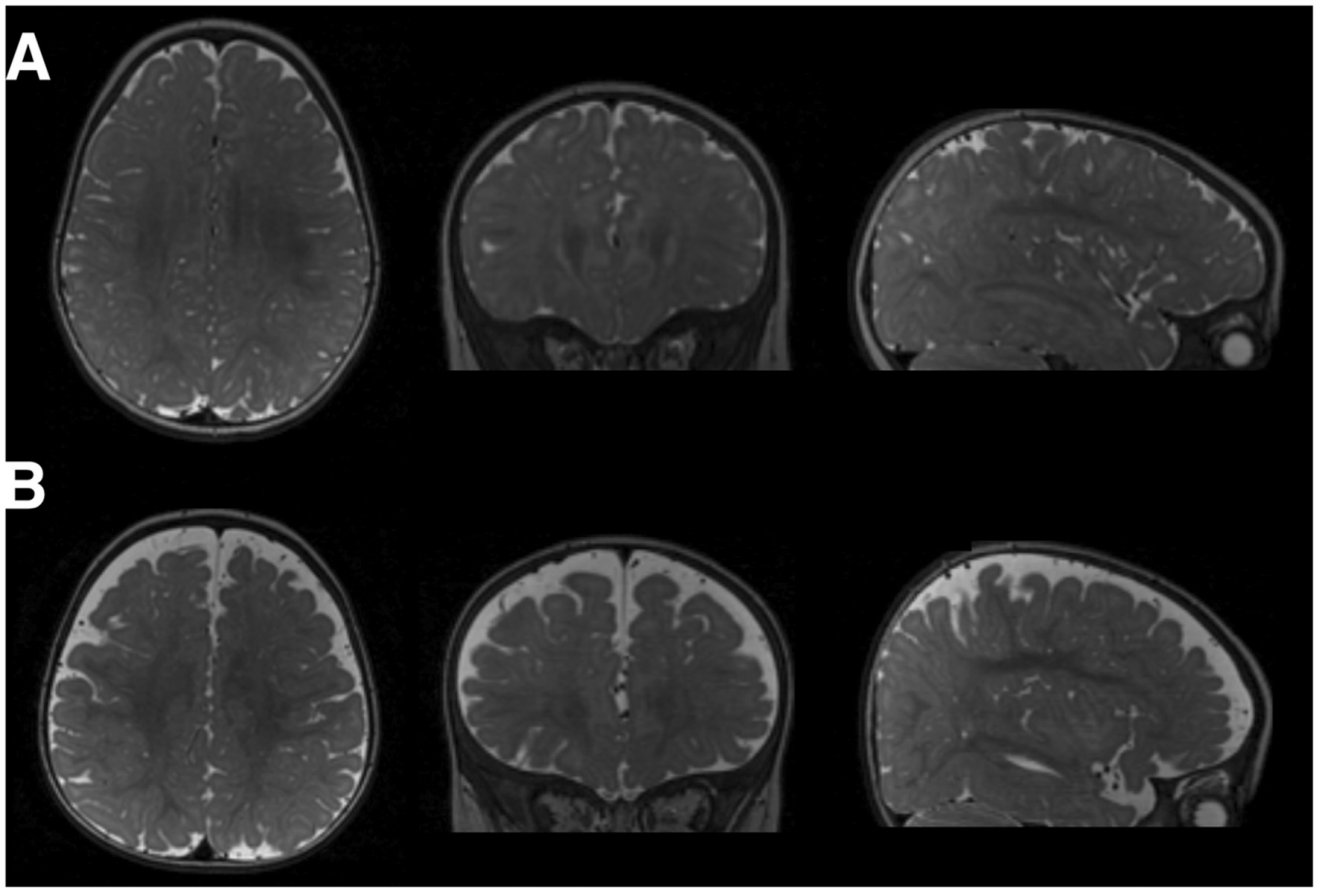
MRI image showing (A) normal 6-month-old's brain and (B) 6-month-old's brain with extra-axial CSF. Shen, *J Neurodev Disord*, 2018.
